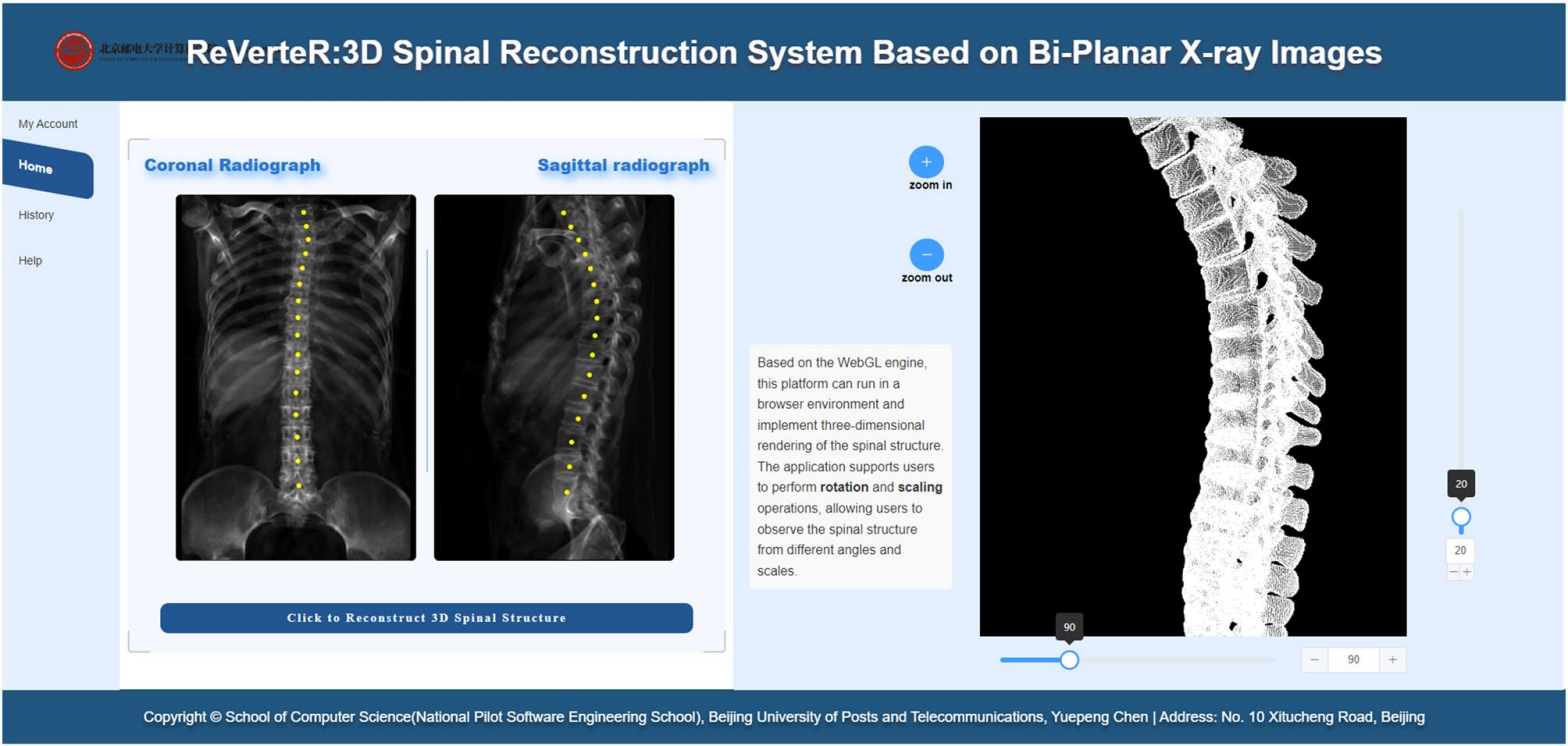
Interactive interface developed based on our proposed clinical deployment-oriented 3D spinal reconstruction framework, where automatically centroid-annotated bi-planar radiographs and reconstructed 3D shape are both presented. A series of customized functions are also integrated.
